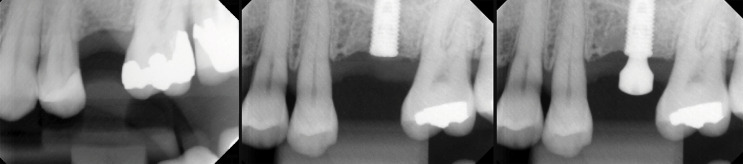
Standardized radiographs for measuring crestal bone loss. Left image - initial (edentulous area without implant); center image - after implant placement; and right image - after osseointegration of the implant.
